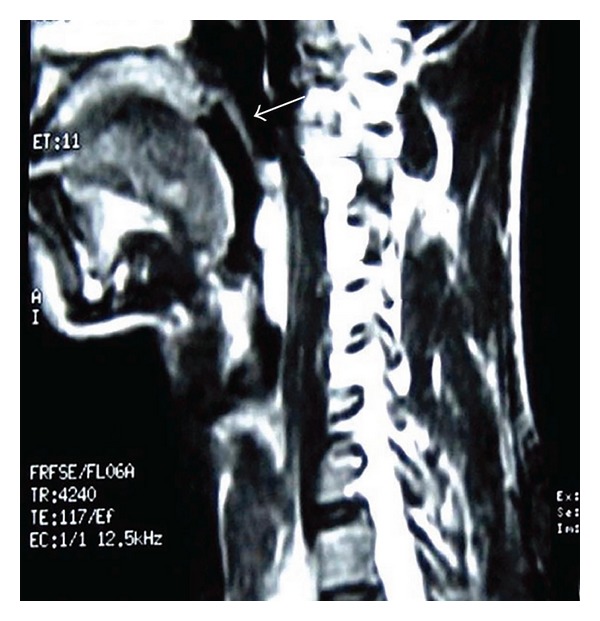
A T2-weighted MRI sagittal image showing the tonsillar polyp with the stalk arising from the inferior pole of the tonsil and extending posterior to the epiglottis and into the postcricoid region with partial occlusion of the glottis.
